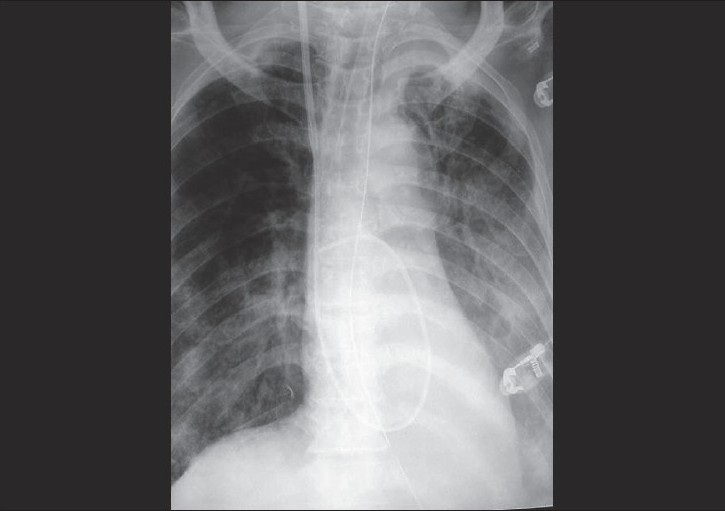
Chest radiograph showing bilateral infiltrates with pulmonary artery catheter
